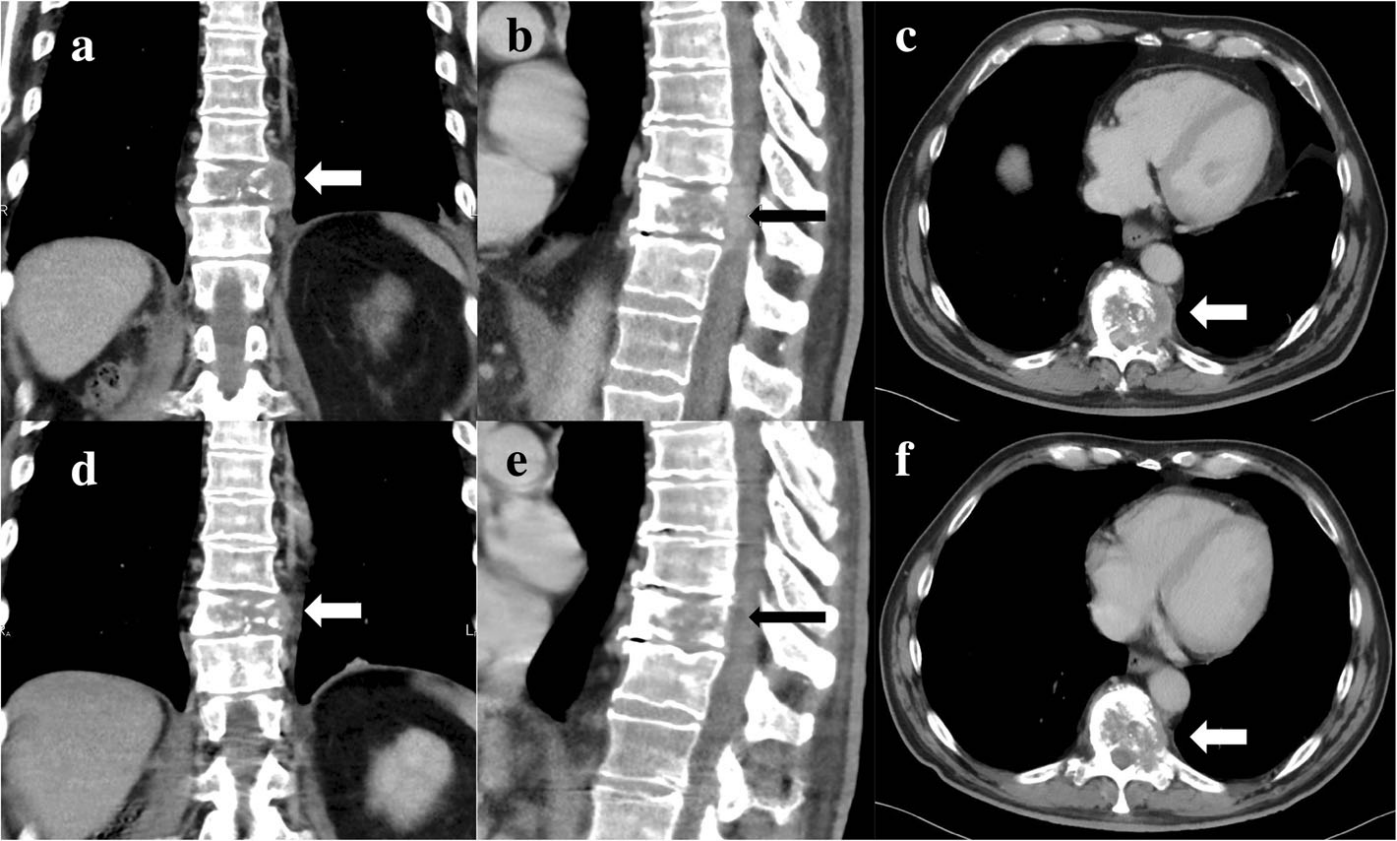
Frontal, sagittal, and axial computed tomography scan demonstrating a destructive mass affecting Th11body (see arrows) from April 2016 (a–c) and August 2016 (d–f)
